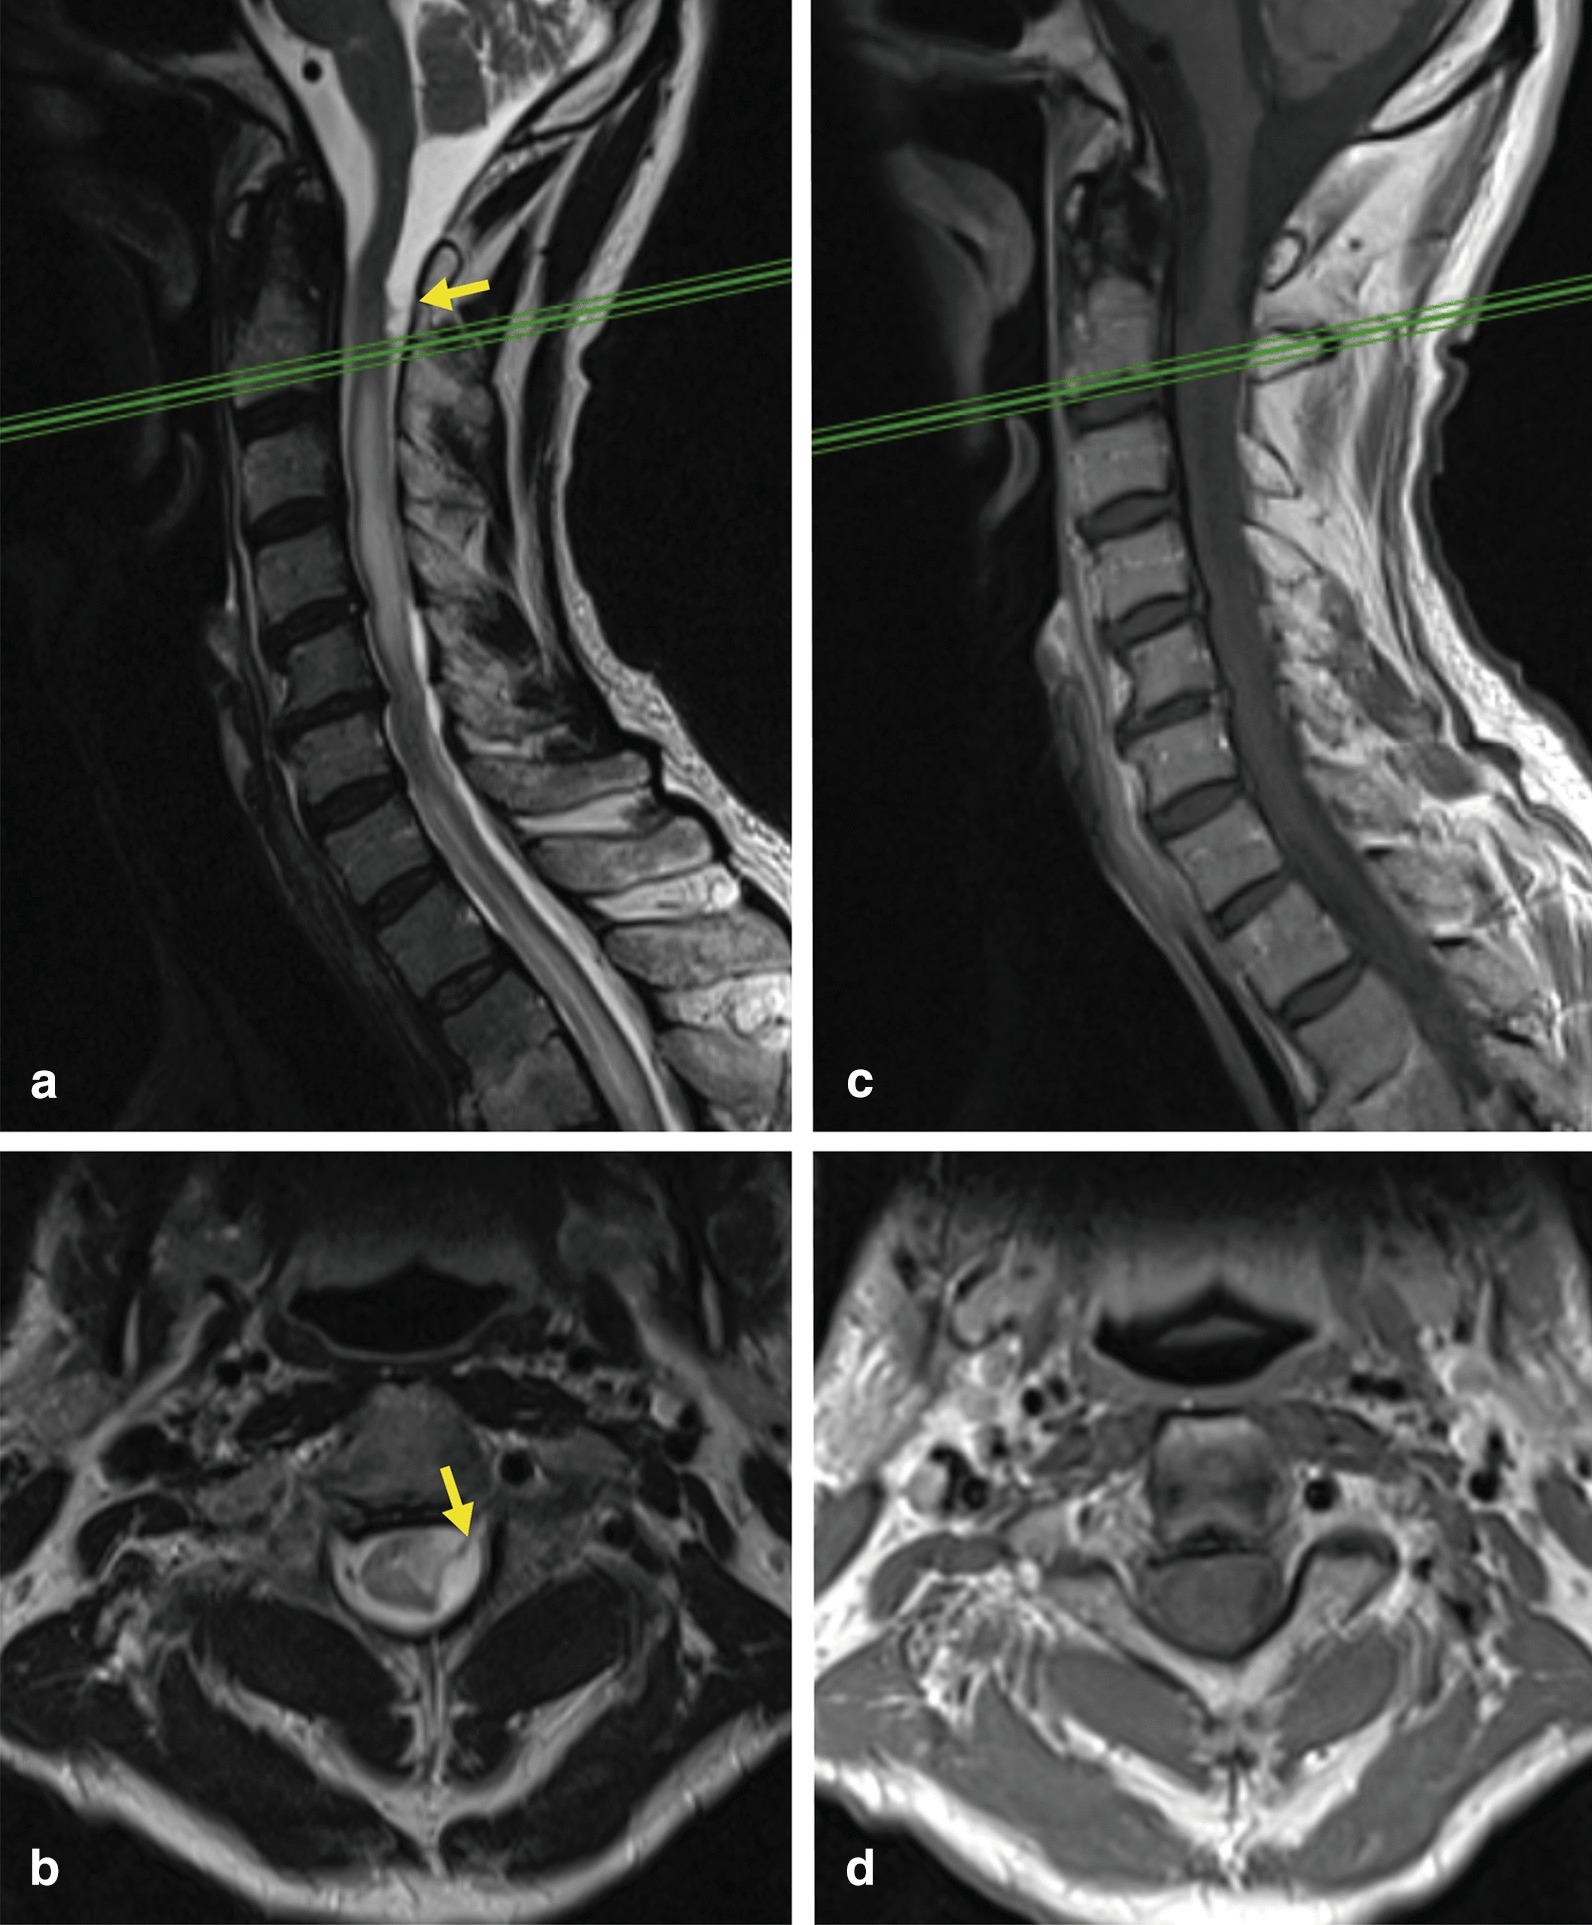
a, b T2-weighted magnetic resonance imaging (1.5 T, 3 mm slice thickness) 12 months post-injury demonstrated the development of arachnoid adhesions at C1/2 (yellow arrow) and medullary edema at the cervical and upper thoracic level. As an incidental finding, a Th2/3 fracture occurred at the site of the hemangioma. c, d Following contrast enhancement, an underlying pathology could be excluded
